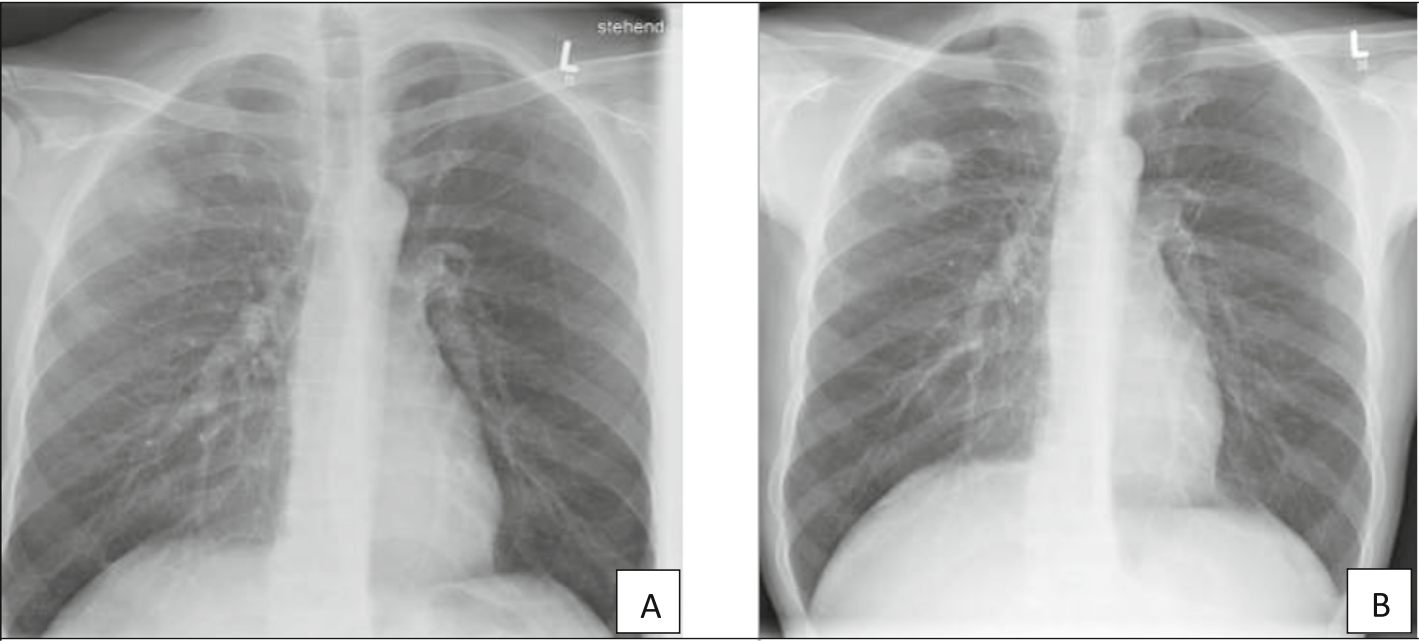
chest X-rays performed in January (a) and February (b) documenting the formation of a cavitary lung lesion over the time span of 6 weeks
